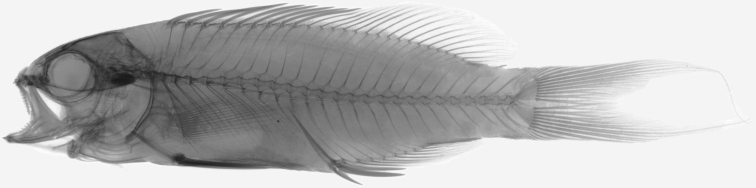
Radiograph of holotype, CAS 247252. Photo by J Fong.
